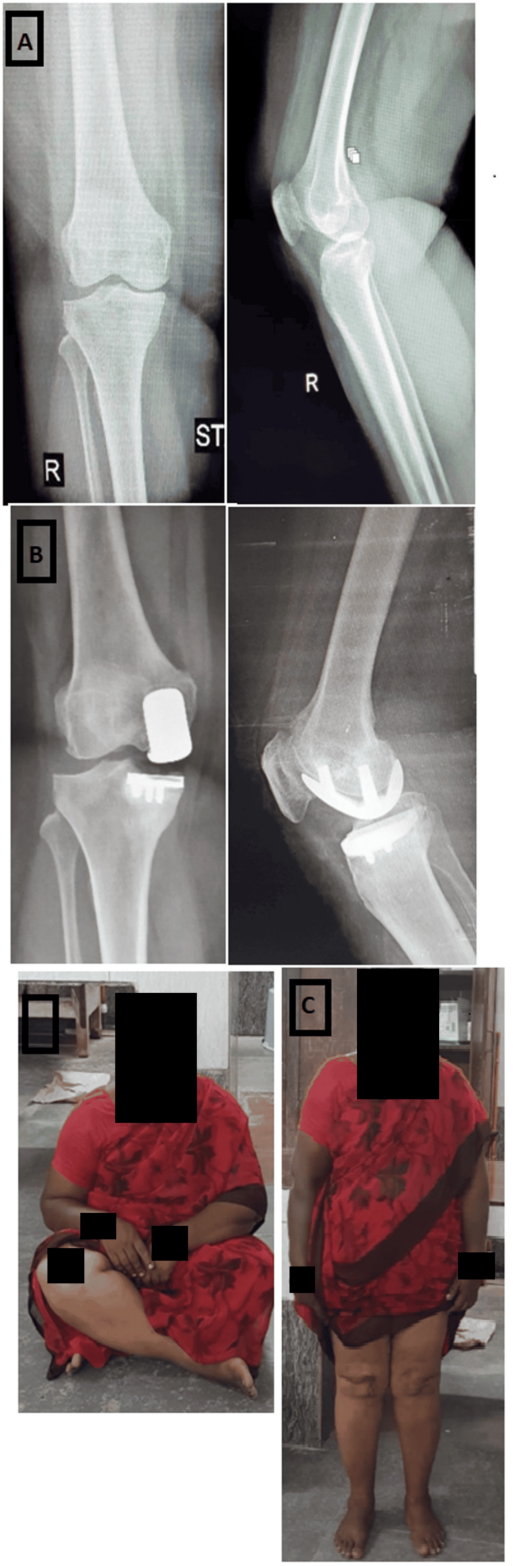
Radiographic and clinical assessment of a 50-year-old woman with severe medial knee pain (A) Pre-operative standing radiographs showing grade 3 medial osteoarthritis. The limb alignment before surgery was 10 degrees of varus. (B) Post-operative radiographs taken at a two-year follow-up. (C) The patient is now pain-free, with excellent knee function, and the radiographs show a stable and well-fixed implant. Importantly, there are no signs of progression of lateral joint space narrowing.
